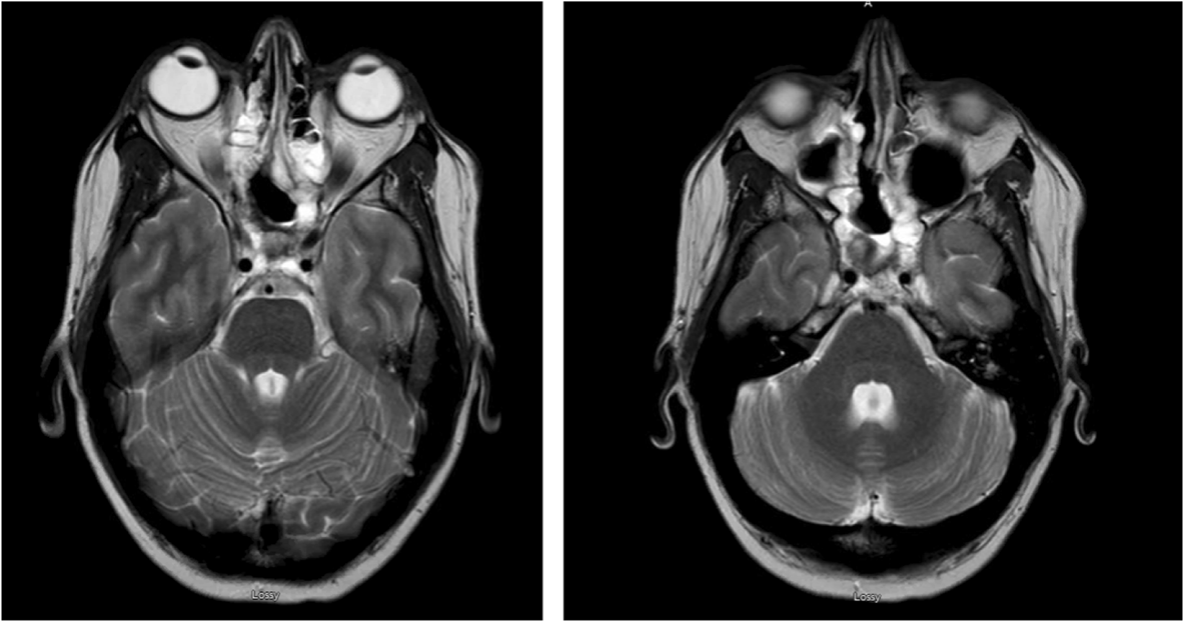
Magnetic resonance imaging demonstrating significant primary tumor response 2 months after resection and status post-initial chemoradiation therapy (+ 69 days from Fig. 1)
